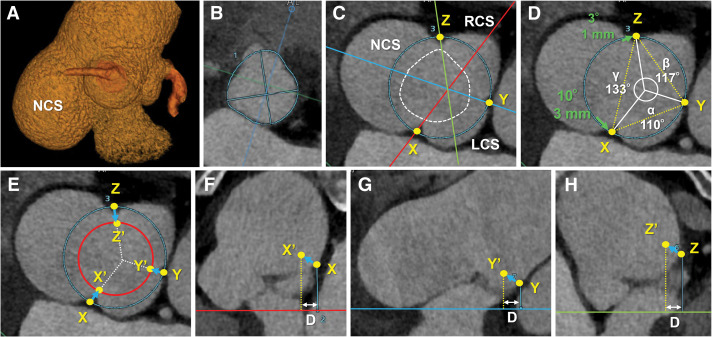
Preoperative CT assessment of the aortic root. (A) 3D CT images showing the SVA located at the NCS. (B) Multiplanar reconstruction at the VBR plane through the 3 nadir points of the LCC, RCC, and NCC. The blue curved line traces the VBR for area and perimeter calculation. (C–E) Multiplanar reconstructions at the commissural plane. The blue circle represents the commissural circle. Yellow points X, Y, and Z indicate commissural positions. (C) White dotted line overlaps the VBR. Red, blue, and green lines represent cutting planes connecting the center of the aortic valve (coaptation point) to commissures X, Y, and Z, respectively. (D) ICDs are shown as yellow dotted lines. Cusp angles α, β, and γ correspond to the LCC, RCC, and NCC, respectively. Green arrows indicate the horizontal shift of commissures from the 120° even positions. (E) Red circle represents the graft contour. Yellow points X′, Y′, and Z′ indicate predicted commissural positions after aortic root reimplantation. Cusp angles are preserved postoperatively. (F–H) Sagittal views along the red (F), blue (G), and green (H) cutting planes (from panel C), perpendicular to the VBR plane. “D” denotes the difference in radius between the estimated VBR and the commissural circle. Predicted CH is calculated as CH ≈ √(D^2^ + measured CH^2^). CH, commissural height; ICD, intercommissural distance; LCC, left-coronary cusp; NCC, noncoronary cusp; RCC, right-coronary cusp; SVA, sinus of Valsalva aneurysm; VBR, virtual basal ring
